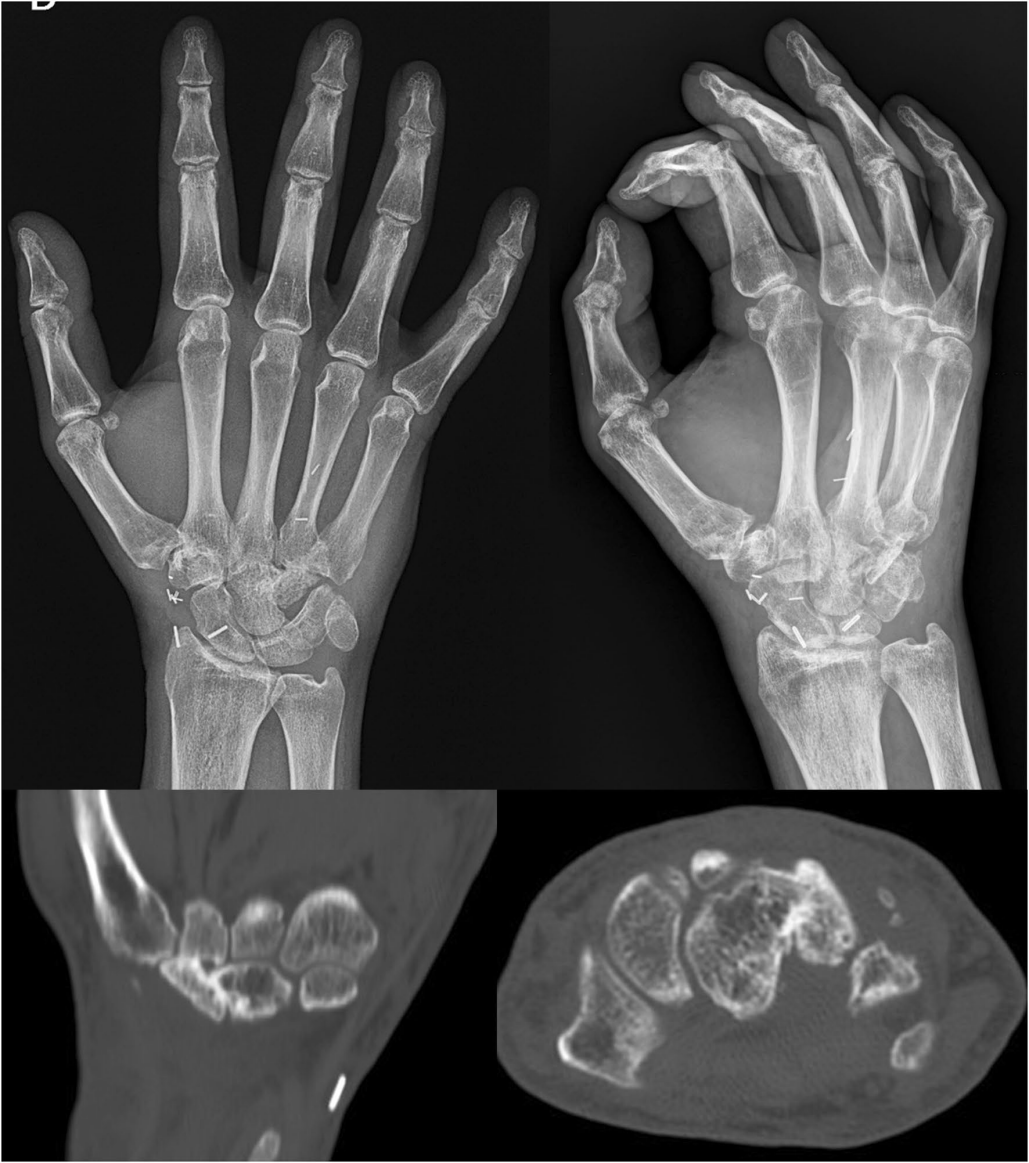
X-ray and CT scan, 16 weeks and 6 months postoperatively. Flap viability and ulnar carpal-metacarpal joint stability are evaluated
